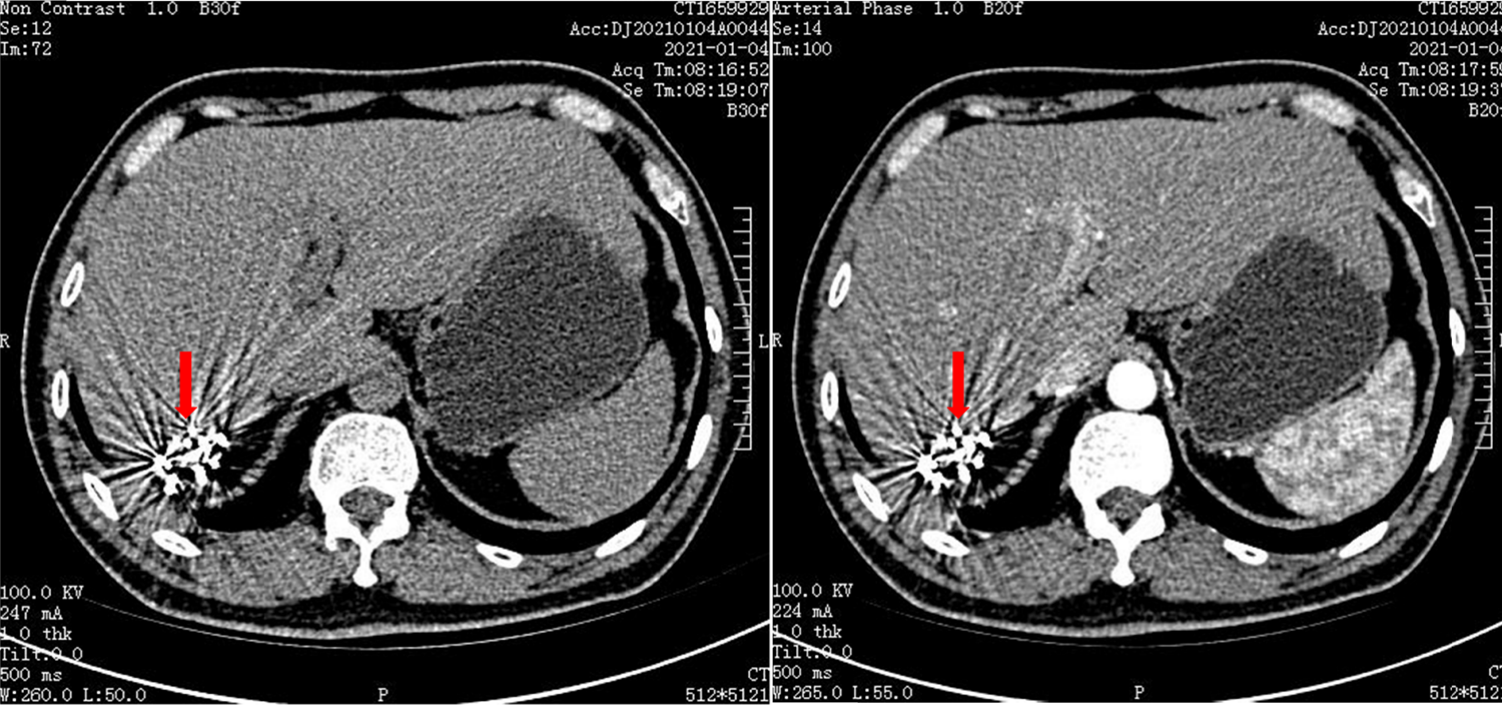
CT-scan showing that lesions were completely necrotic and no signs of recurrence after 5 years of treatment.
